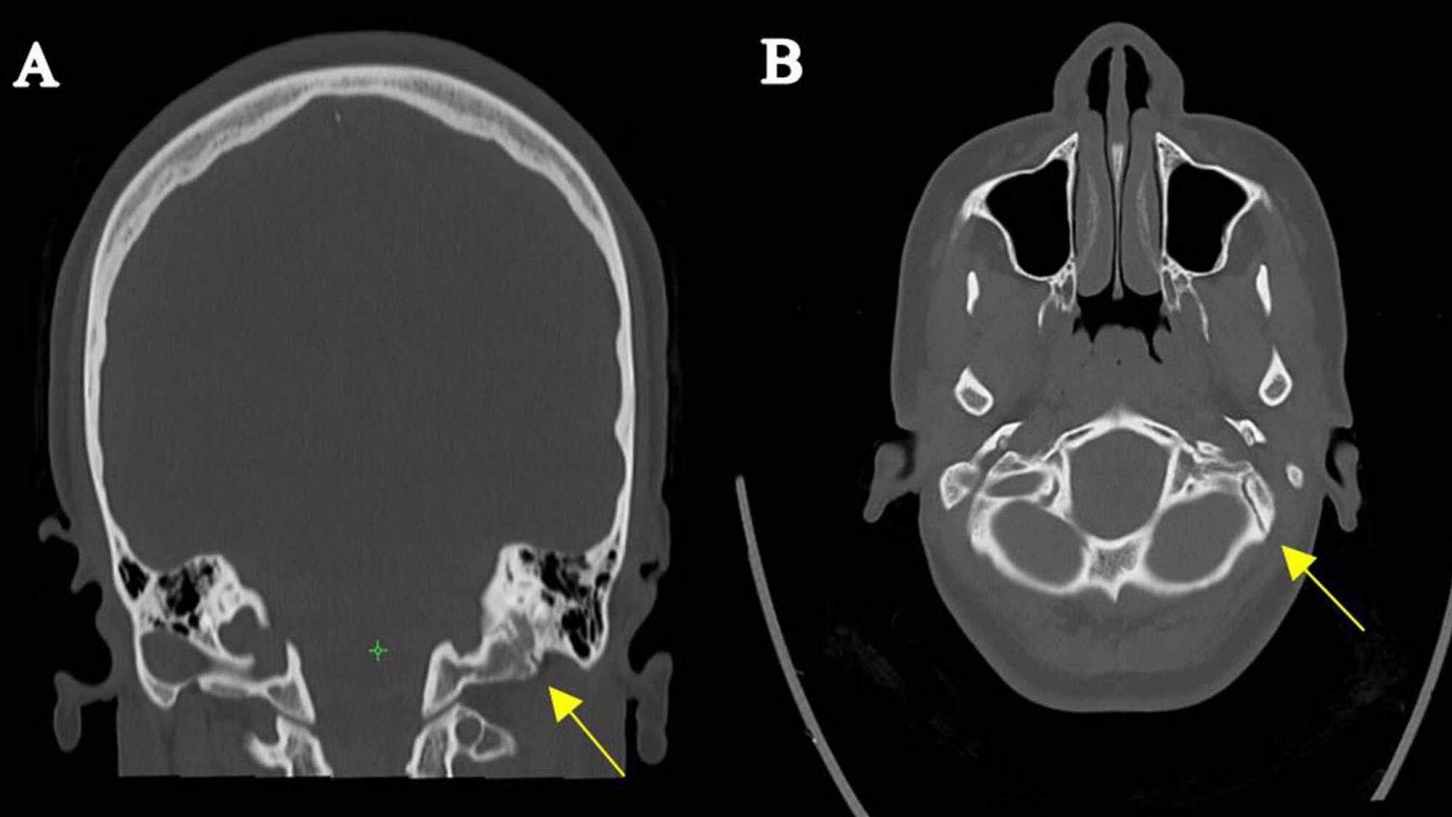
Nonenhanced head CT showing the left petrous bone fracture. (A, coronal view; B, axial view).
